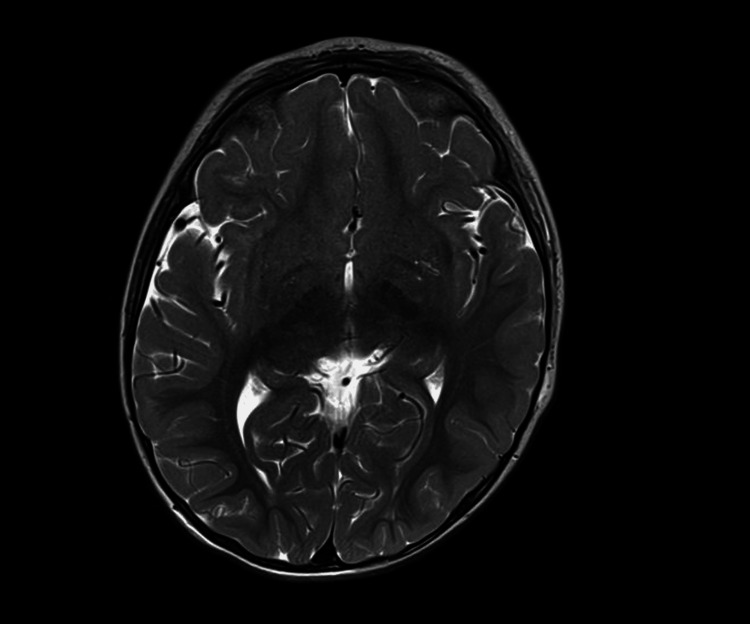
Axial T2-weighted MRI of the brain showing no edema, encephalomalacia, or abnormal signal intensity. No structural or temporal lobe abnormalities were identified.
